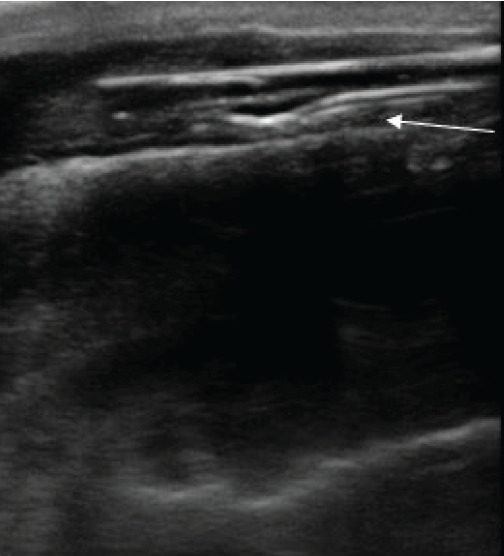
Longitudinal ultrasound view of the penis using a high-frequency linear probe. A hyperechoic linear object (arrow) is seen in the urethra, corresponding with the inserted foreign body.
